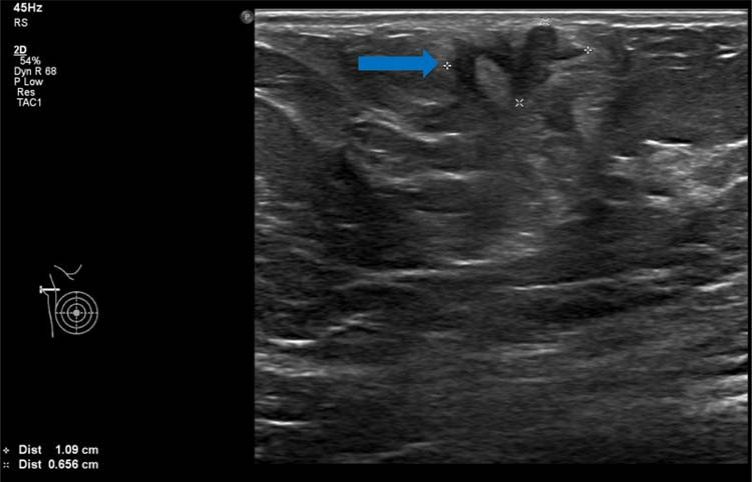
Ultrasound scan of right axillary accessory breast tissue shows focal area of illdefined hypoechogenicity underlying the dermis measuring 10.9 × 8.3 mm (blue arrow). No associated significant hypervascularity. The imaging appearance are indeterminate, U3 (Royal College of Radiologist, Breast Group Classification).
